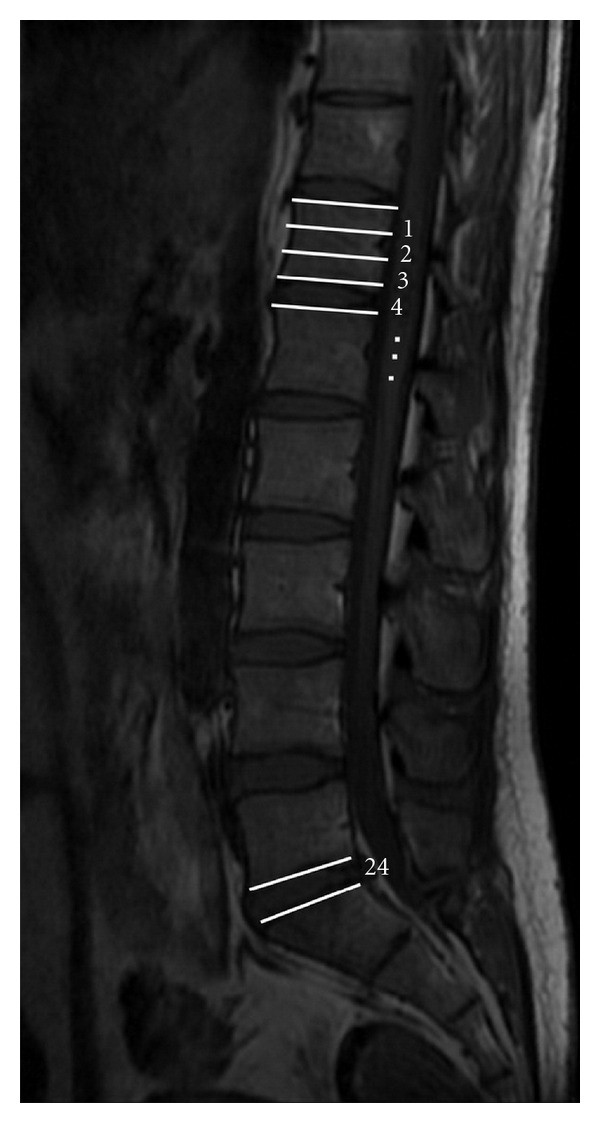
Mid-sagittal T1-weighted MRI of lumbosacral spine. Each vertebra is divided into 3 equal parts of upper, middle, and lower segments. Vertebral segments and disc spaces were numbered consecutively from T12 upper segment downward to L5S1 intervertebral disc.
